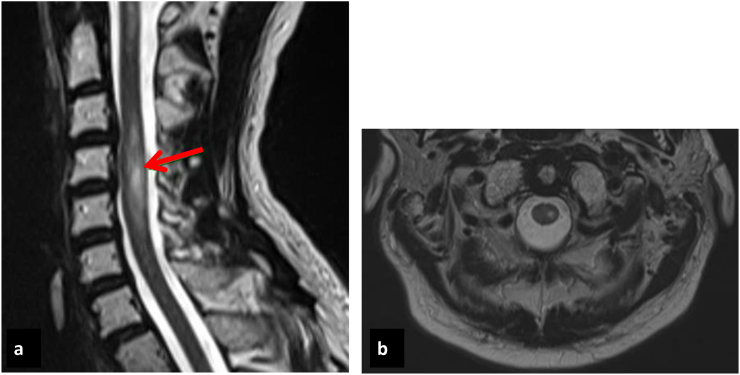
Axial (a) and sagittal (b) contrast magnetic resonance image findings of C1 and C3-5 using the T-2 weighted sequence.
